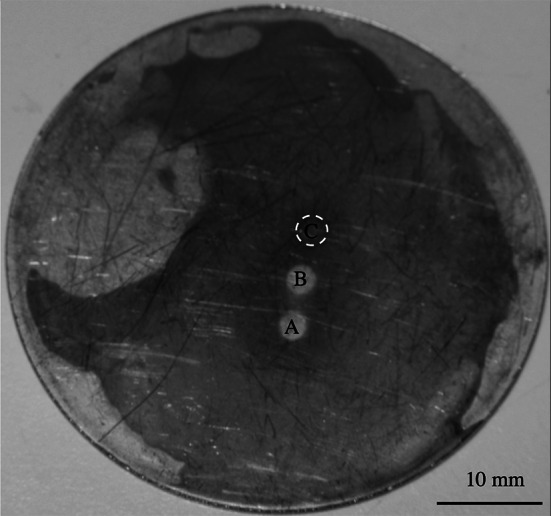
Photograph of a lard layer after 90 min immersion in CTAB at pH 10.4, 50 $$^\circ $$C, with *H* set as 100 mm. *A*, *B* and *C* mark locations gauged by the sFDG device. *A* and *B* were gauged twice as often as *C*
